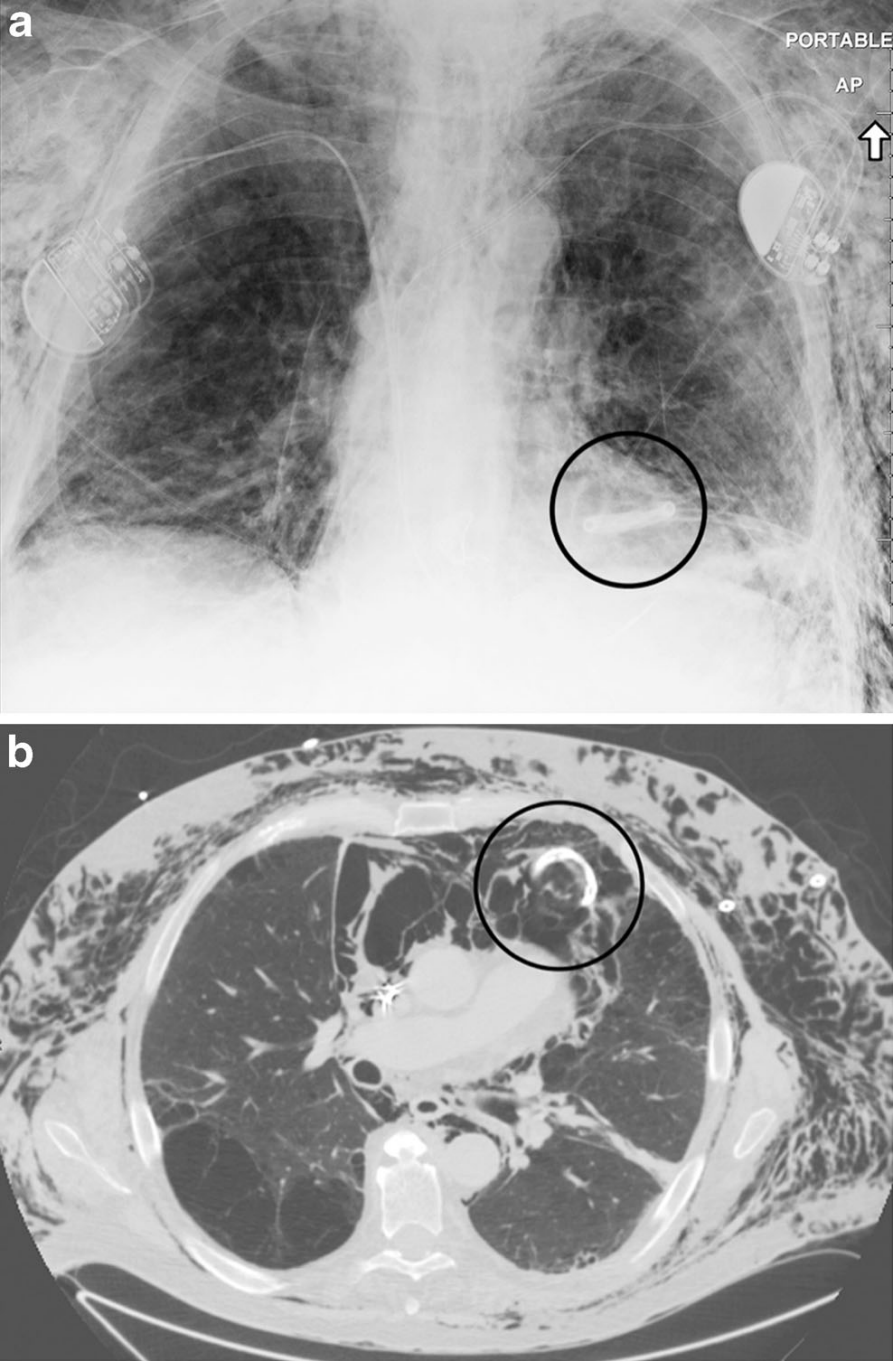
A 67-year-old male with multiple rib fractures status post fall. Chest radiograph (a) demonstrates a pleural pigtail drain projecting over the lower left chest (*circle*). Axial CT image through the upper thorax (b) demonstrates anterior mediastinal location of the drain (*circle*) with extensive pneumomediastinum and chest wall emphysema
